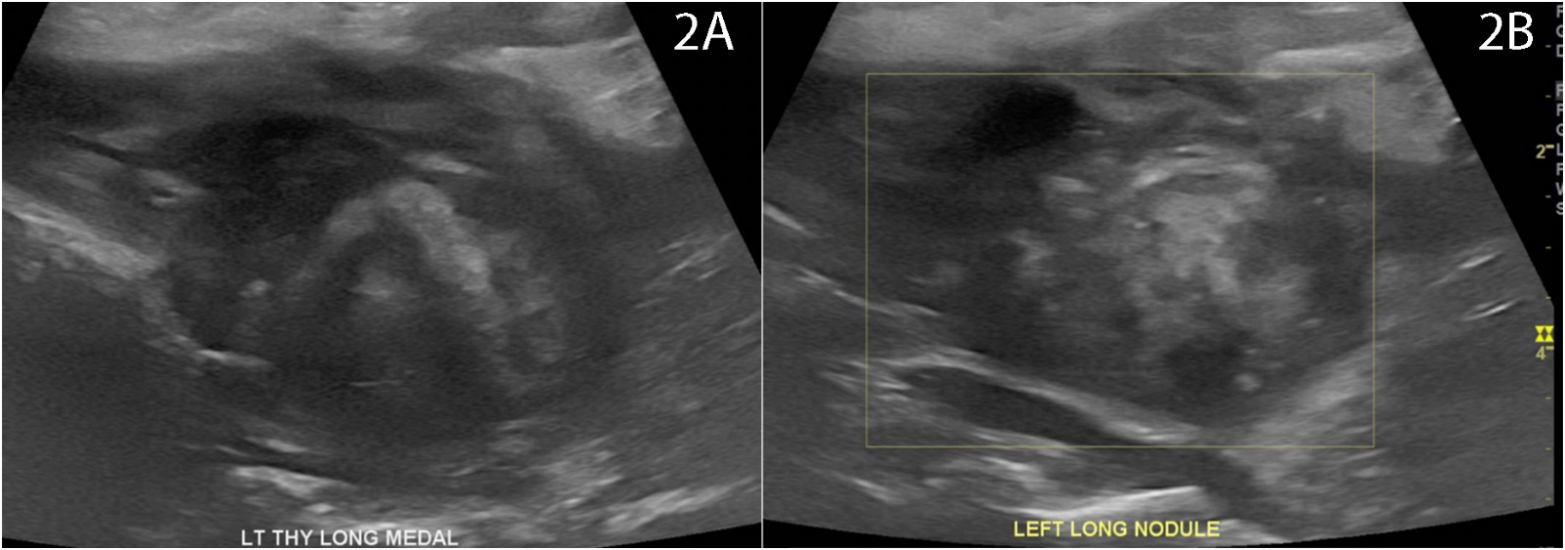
Photograph of patient neck at (A) 7 days post-RFA at time of initial complaint of transient redness and discomfort with spontaneous recovery and (B) at time of recurrent symptoms of pain, swelling, and redness at 1 month post-RFA prompting CT neck demonstrating findings of nodule rupture.
